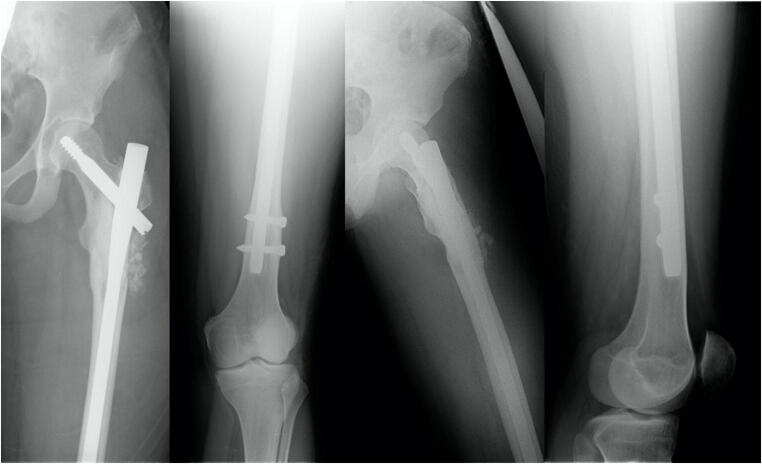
Anterior-posterior and axial X-ray of the left femur after spongioplasty
